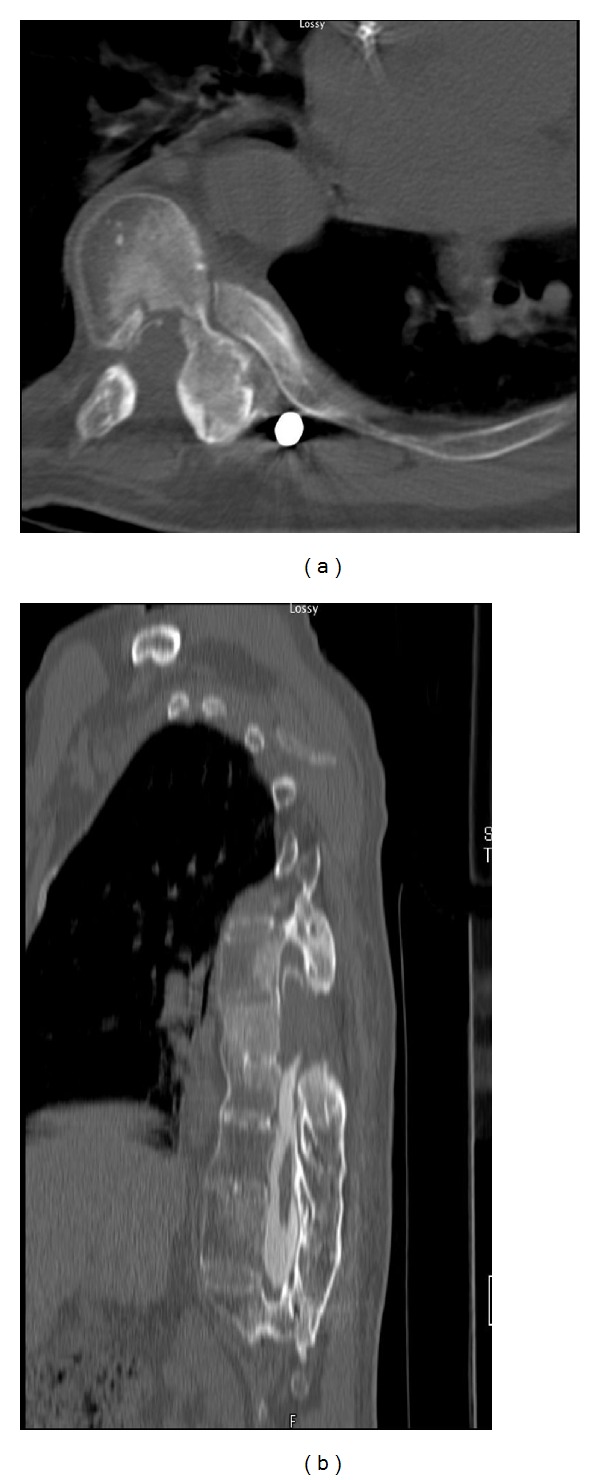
Axial and sagittal reformatted CT myelogram images over a year after the initial resection reveal tumor recurrence at the laminectomy segment with contrast block.
